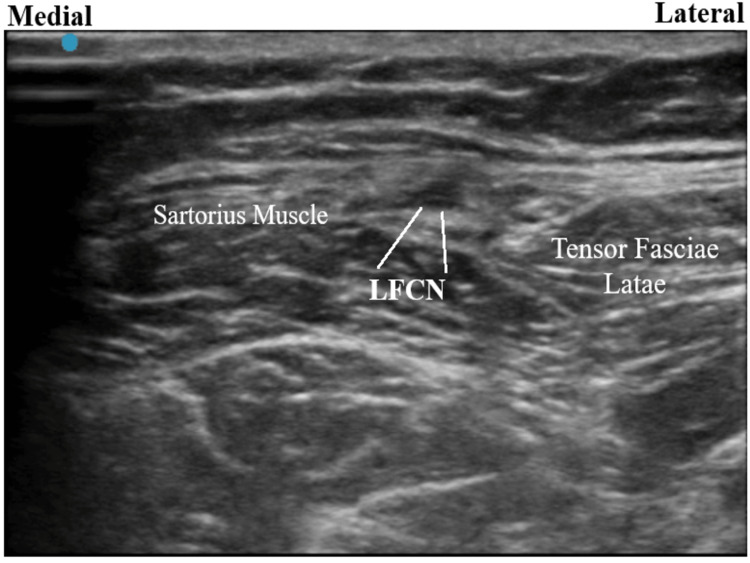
Ultrasound image of the lateral femoral cutaneous nerve
